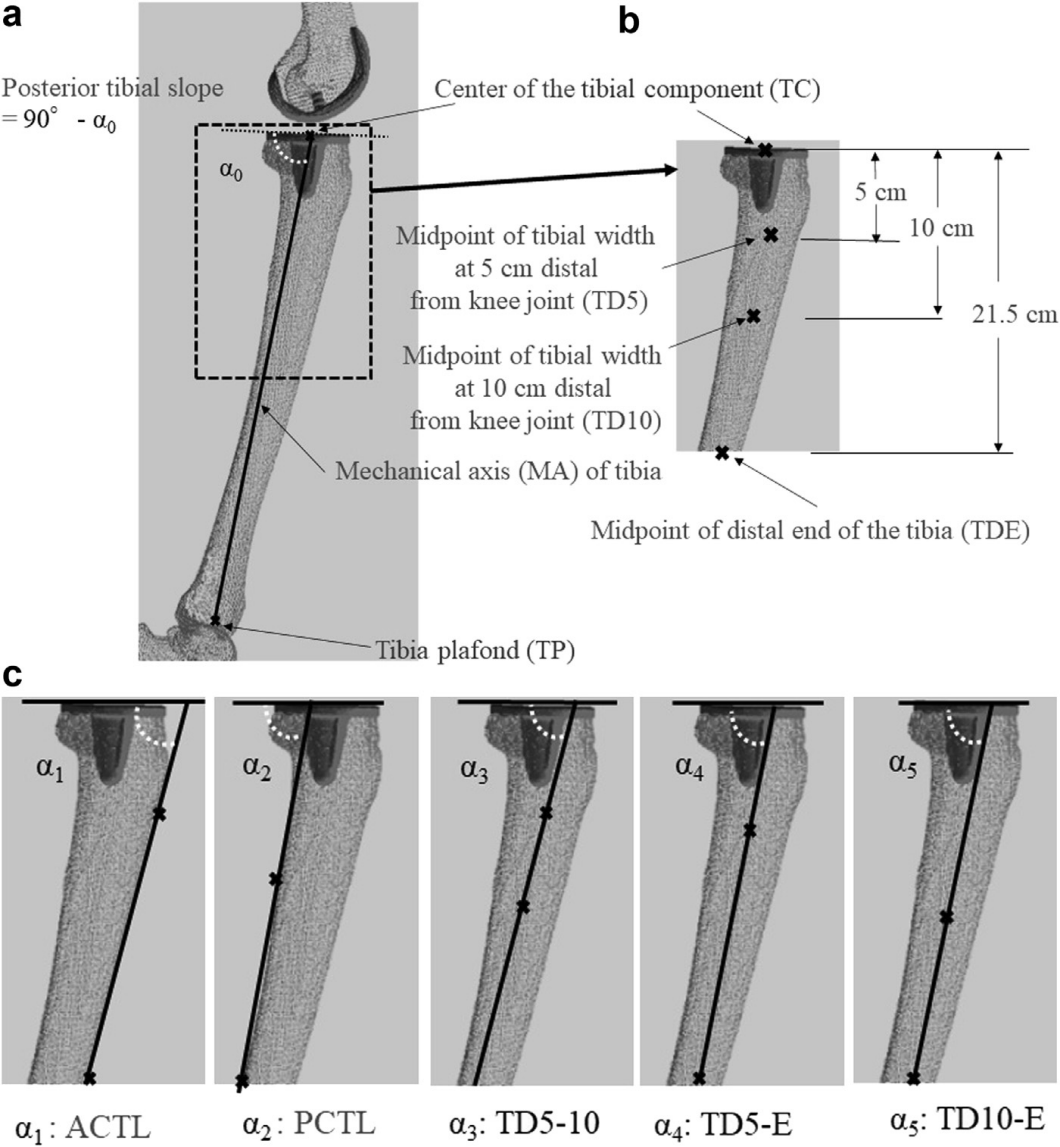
Cross-sectional views from the 3-dimensional digital model of the tibial prosthesis and bone complex in the sagittal plane are shown. The images, including the full-length lateral tibial radiograph, were used as FLTR (a), and the distal half of the lateral standard knee radiographs (36 × 43 cm) were used as LSKR (b). Posterior tibial slope was measured with reference to the sagittal MA (a), ACTL, PCTL, TD5-10, TD5-E, and TD10-E (c). ACTL, anterior cortical tibial line; PCTL, posterior cortical tibial line.
